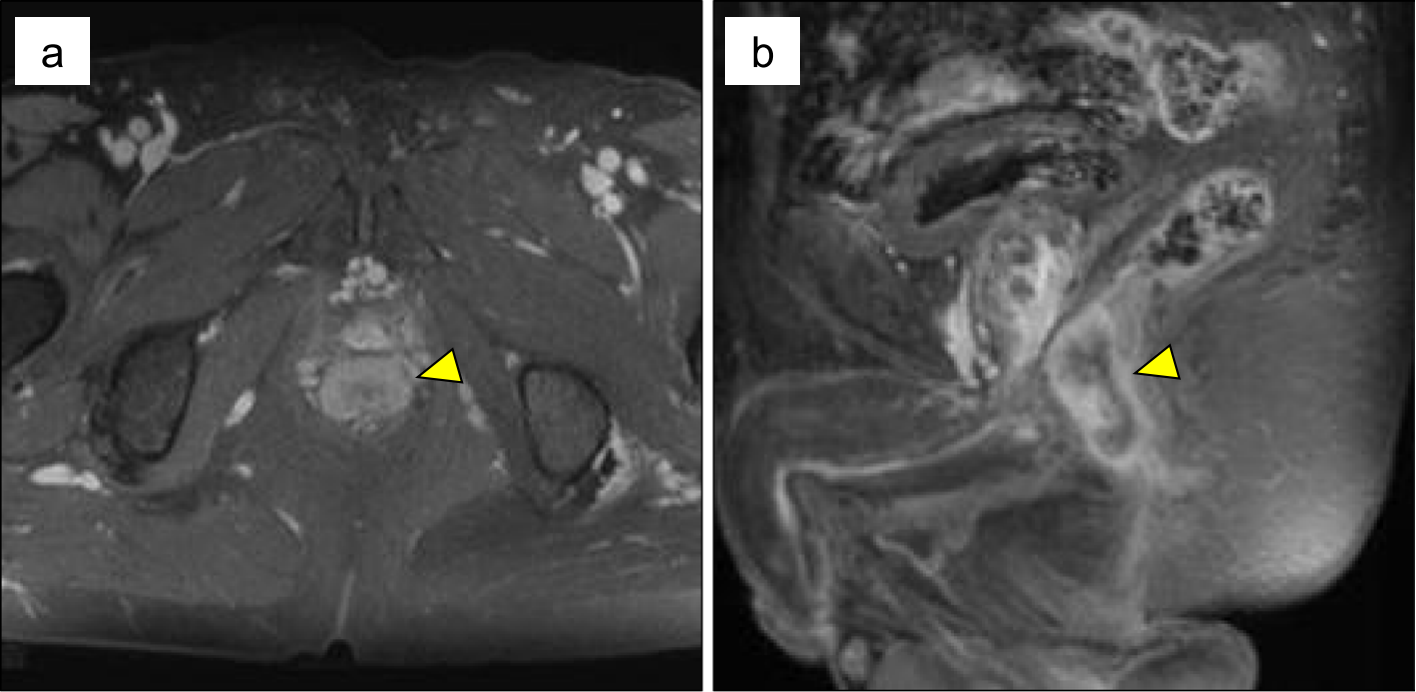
Pelvic magnetic resonance imaging scan demonstrated the extramural growth of the tumor on the ventral side of the rectum. There was no invasion of the prostate. a Axial image. b Sagittal image
